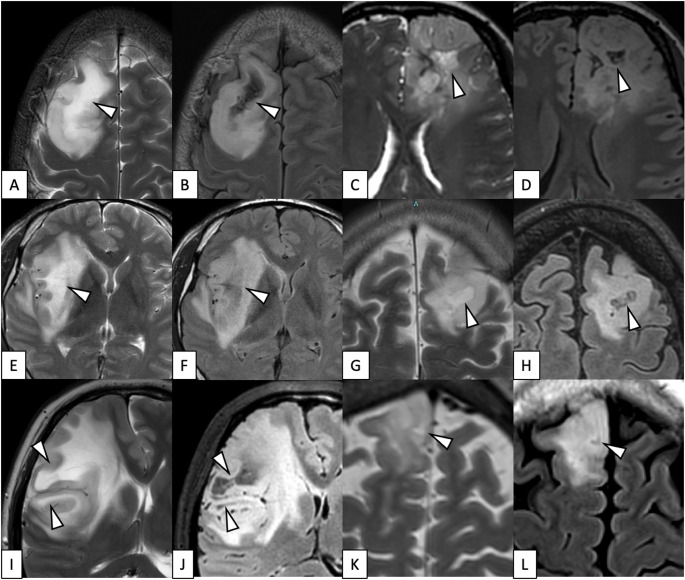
Example cases of subcortical fluid-attenuated inversion recovery (FLAIR) signal drop (scFLAIR-D) in oligodendroglioma, IDH-mutant and 1p/19q-codeleted. T2-weighted images (A, C, E, G, I, K) and FLAIR images (B, D, F, H, J, L) are demonstrated in each case (A and B: 33 year-old woman, CNS WHO grade 3; C and D: 44 year-old woman, CNS WHO grade 3; E and F: 50 year-old man, CNS WHO grade 3; G and H: 35 year-old woman, CNS WHO grade 3; I and J: 31 year-old woman, CNS WHO grade 2; K and L: 56 year-old woman, CNS WHO grade 3). FLAIR-hypointense signal corresponding to T2-hyperintense signal with FLAIR signal drop is observed in the subcortical area (arrow heads)
